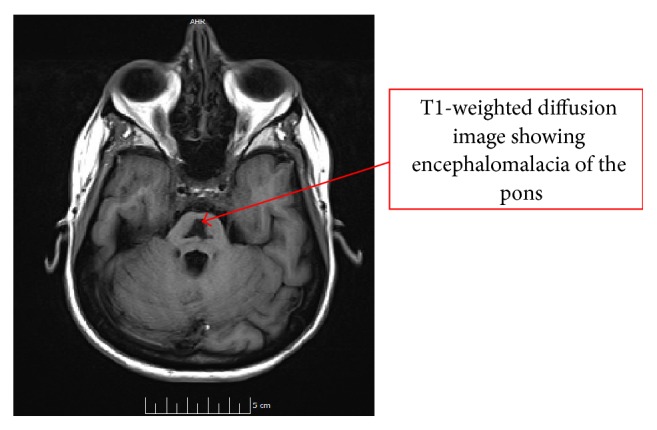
Repeat MRI of brain after recovery.
